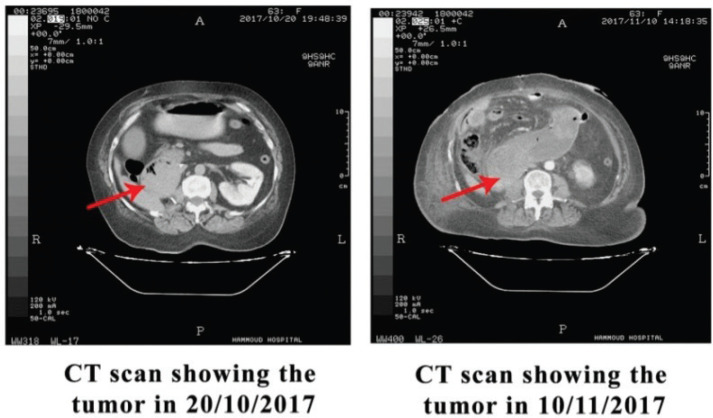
CT scan showing rapid tumour progression.
